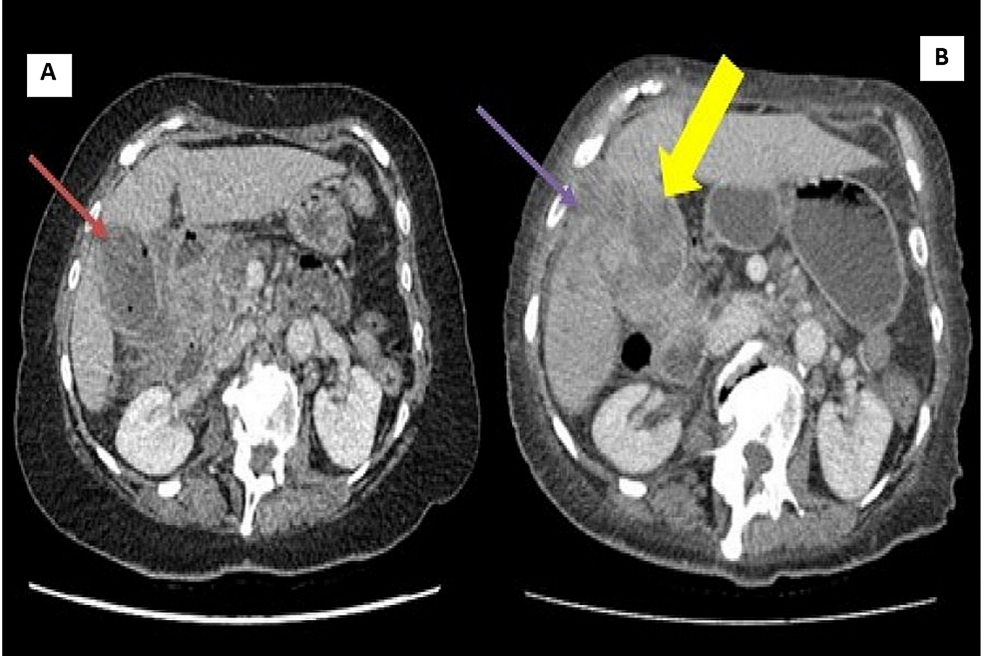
(A) CT of the abdomen with portal venous phase, showing the initial presentation with cholecystitis: thick-walled gallbladder (red arrow) containing gas locules with pericholecystic fat stranding and fluid. (B) CT of the abdomen with portal venous phase, showing the later presentation with extensive wall thickening of the gallbladder (yellow arrow) and inflammation into the adjacent liver (purple arrow). CT, Computed tomography.
